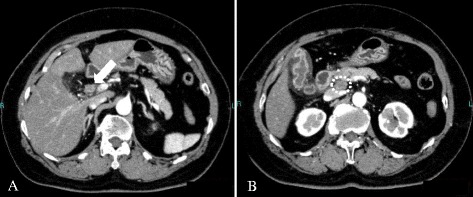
CT findings. Abdominal enhanced computed tomography showed an enhanced tumor located in the hilar bile duct to the left hepatic duct (a: *arrow*). The encircled area indicated the regional lymph node metastasis located along the common bile duct (b)
